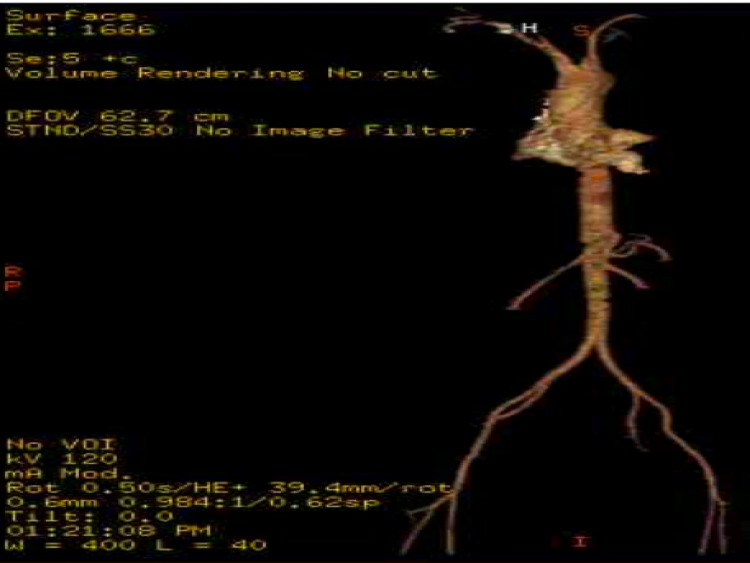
Three-dimensional computed tomography (CT) angiogram of chest, abdomen, and pelvis
